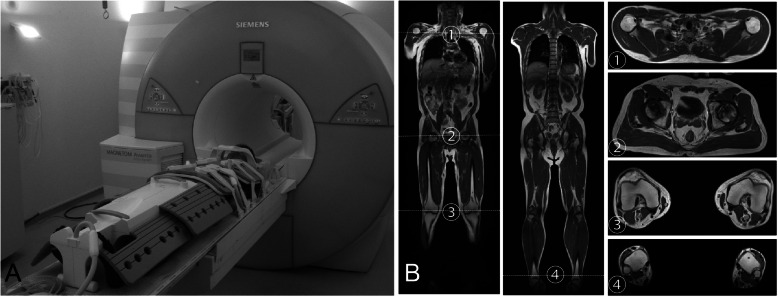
Whole-body MRI. a Set-up of coils (head, chest, abdomen, and extremity coils) in a wide bore magnet device. b Typical coronal and axial images using T1-weighted (T1W) turbo spin-echo images of whole-body MRI. (1) Shoulder joints, (2) hip joints, (3) knee joints, and (4) ankle joints. MRI, magnetic resonance imaging
